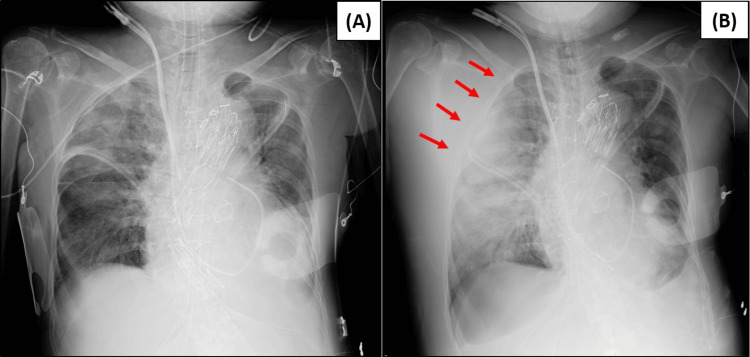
Chest X-rays: Post-scheduled Surgery (Pre-emergency Surgery) Chest X-ray revealed that the right upper thorax was compressed by the large hematoma when compared to the chest X-ray taken immediately after surgery (red arrow). (A) Chest X-ray taken immediately after surgery. (B) Chest X-ray taken after chest wall hematoma was identified.
